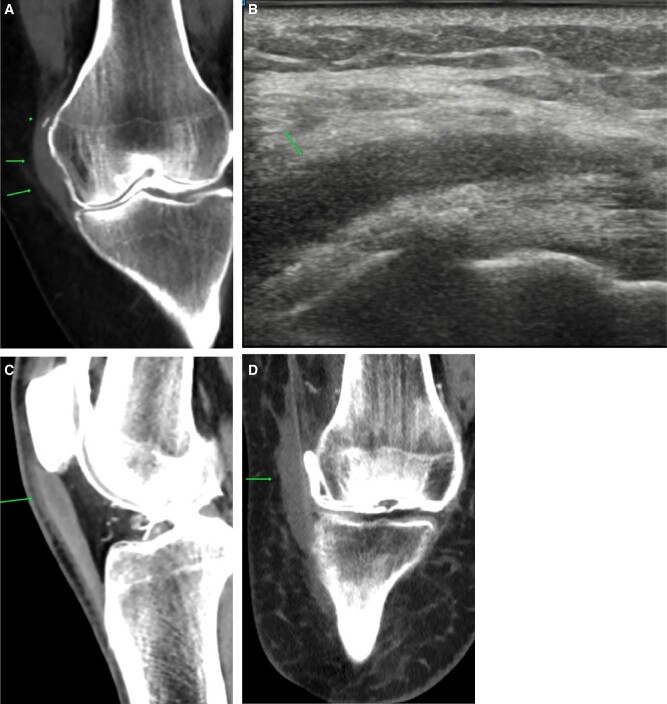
Tendinopathy: (A) MCL calcification and thickening (arrows) in keeping with Pelligrini-Steida disease. (B) Ultrasound image of the same knee treated with ultrasound-guided steroid injection, with the arrow pointing to the steroid + local anaesthetic mixture at MCL bursa. (C) Patellar tendinopathy (arrow) and (D) iliotibial band bursitis (arrow).
